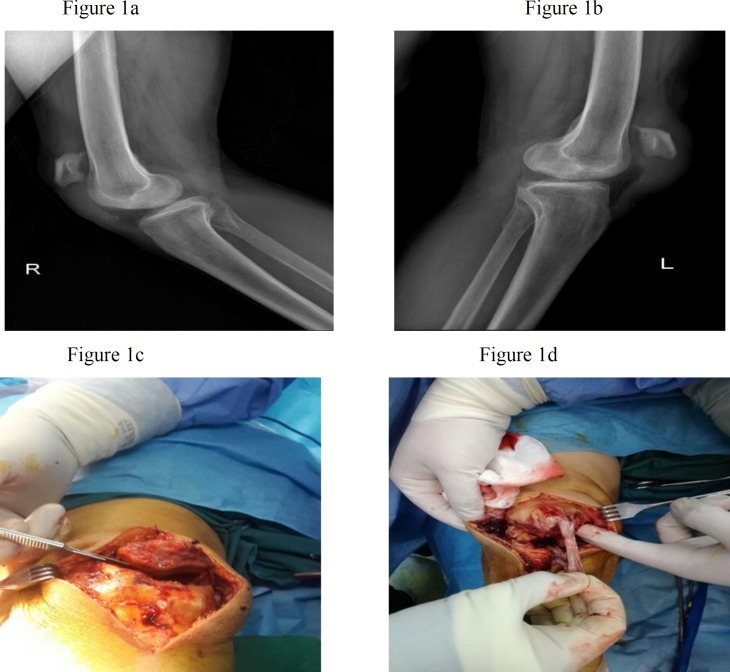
Rt and Lt lateral radiography of knee shows patella Alta, (a) and (b). Intraoperative photography show complete patellar tendon rupture from proximal attachment, (c) and (d)
